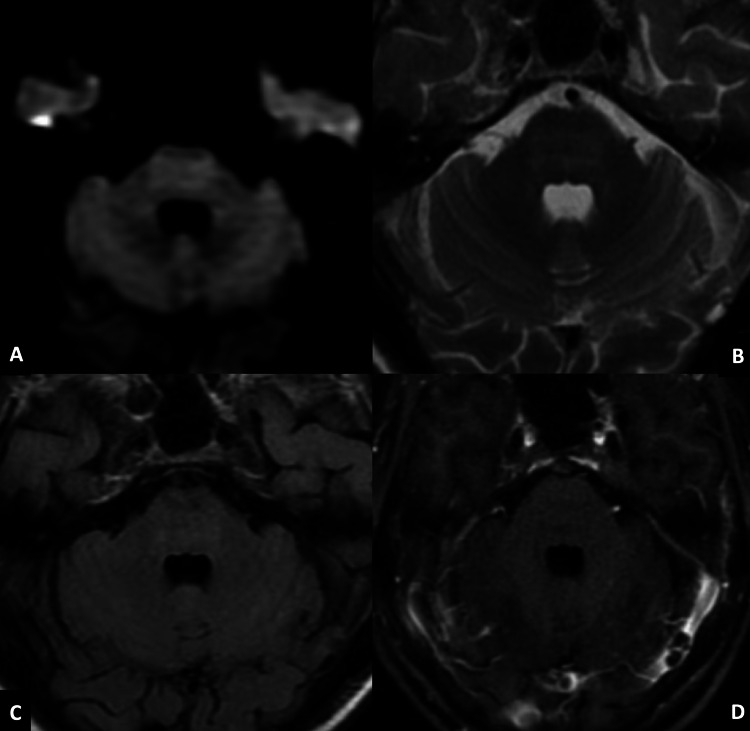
Brain MRI of the patient. Hyperintense signal in central pons with peripheral sparing in axial diffusion-weighted image (A), axial T2-weighted image (B), and axial FLAIR (C). Post-contrast axial T1-weighted image (D) does not show any abnormal enhancement. FLAIR: fluid-attenuated inversion recovery.
